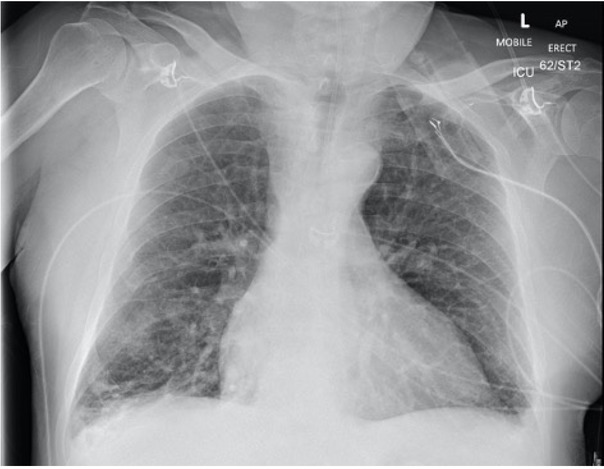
Chest X-ray of Case 1: Bi-basal atelectasis and small bilateral pleural effusion.
